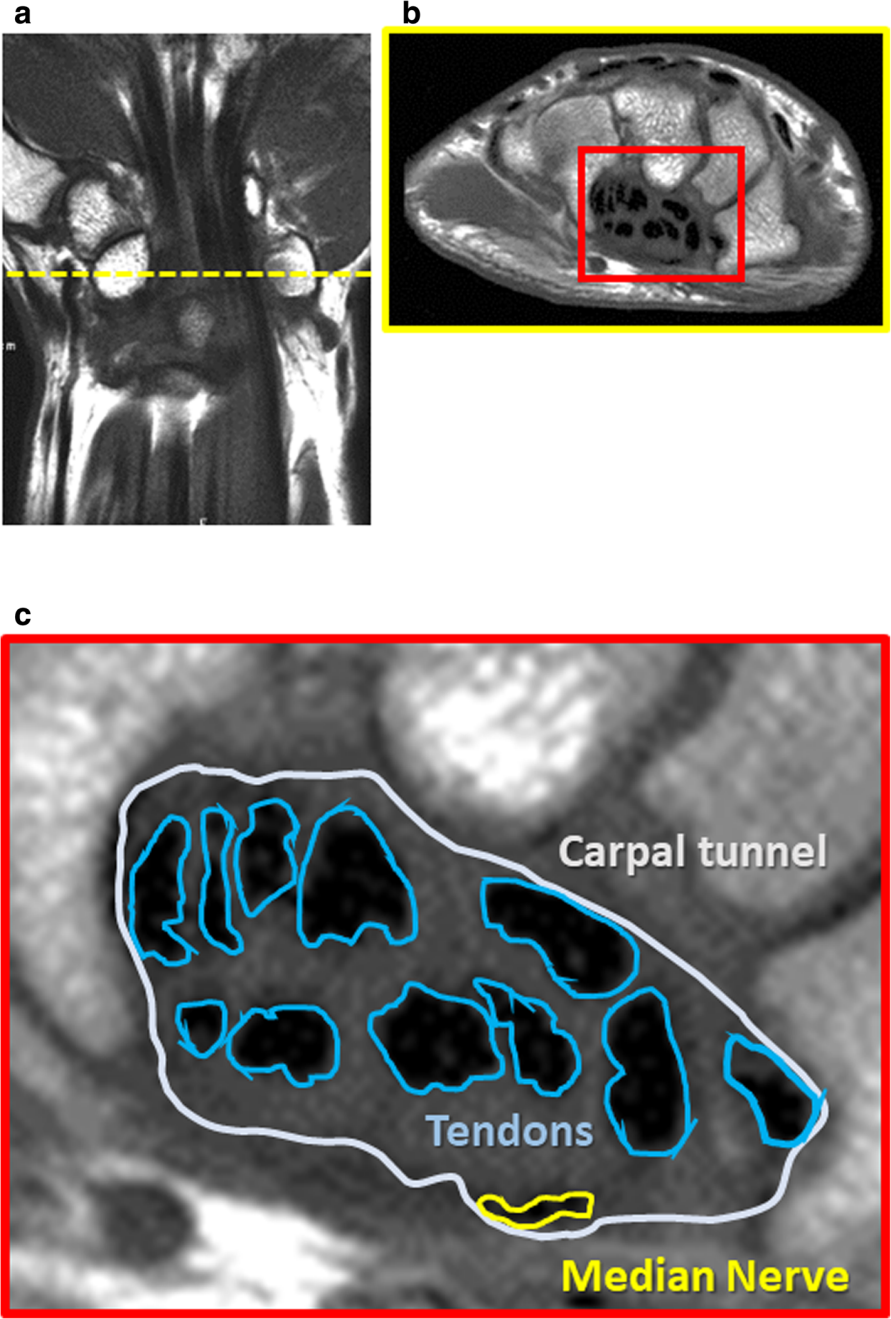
The method of measuring the CSA. In T1-weighted axial image of MRI, at the level of the hook of hamate (a) with the axis of which was vertical to the line from the third web space to the ulnar edge of the palmaris longus tendon (b), the CSA of carpal tunnel, each flexor tendon, and median nerve were calculated (c)
